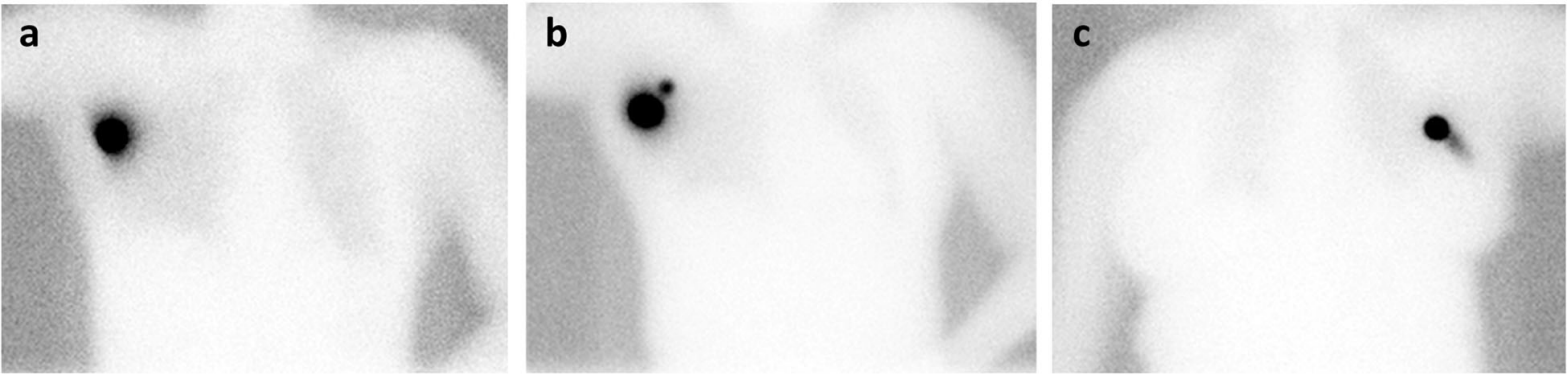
Planar anterior scintigraphy images in three different patients after the ROLL-node injection in the clip-marked lymph node, displaying the three different scintigraphic patterns. a One clear focus is observed in the axilla. b Two focal hotspots are observed in the axilla, corresponding to the ROLL-marked node and drainage to one higher echelon node. c One focal hotspot is observed in the axilla with minimal radioactivity in the needle trajectory.
